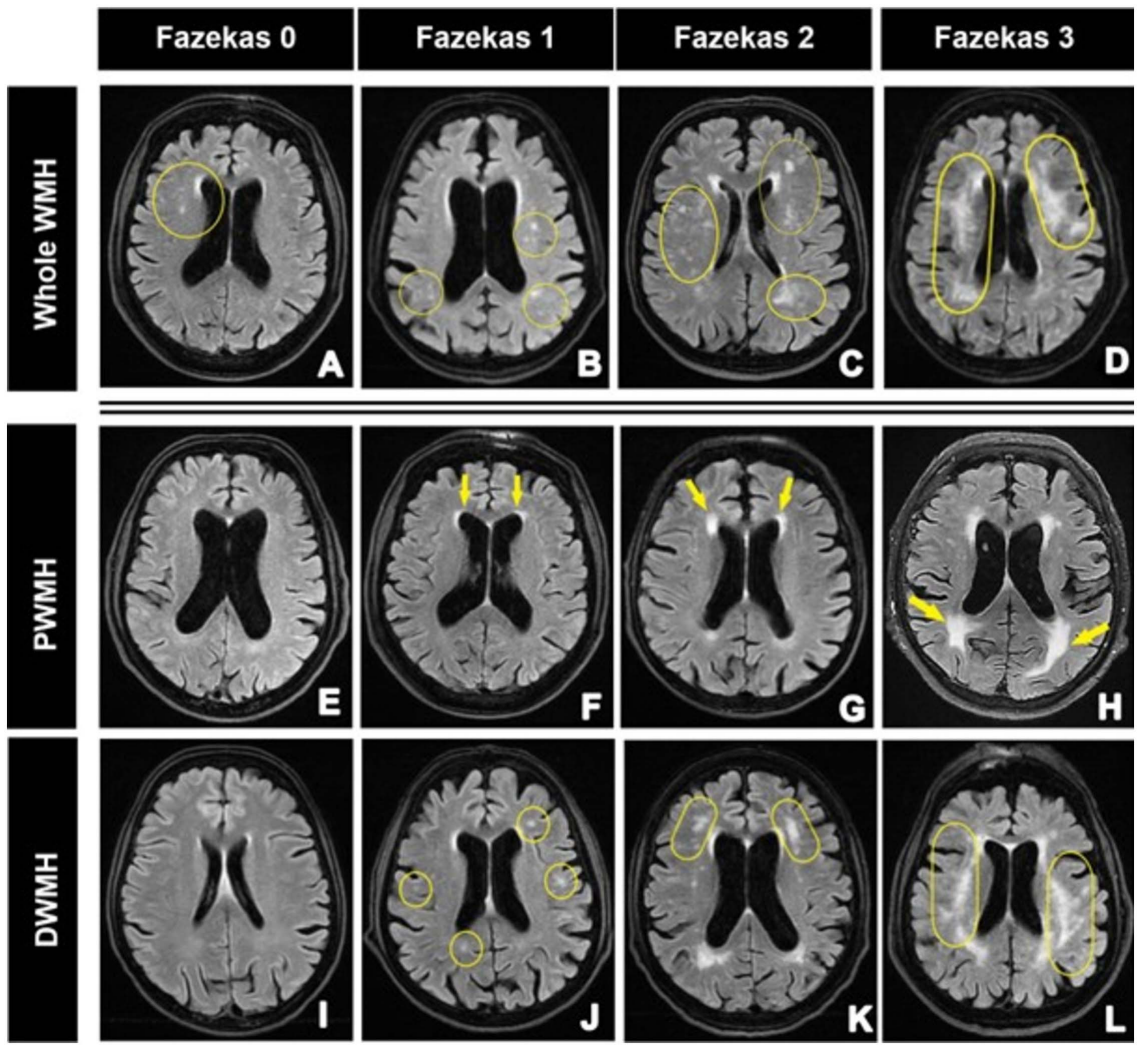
Axial FLAIR images of different patients, mostly with Alzheimer’s disease and vascular dementia clinical presentations (aged between 55 and 87 years) show Fazekas scales. The first row: whole WMHs: (A) single WMH (circled yellow); (B) multiple WMHs (circled yellow); (C) WMHs that start to bridge (circled yellow); (D) large confluent WMHs (circled yellow). The second row: PWMH: (E) absent WMH; (F) “cap and bands” (yellow arrows); (G) smooth periventricular hallo (yellow arrows); (H) PWM extends into DWM (yellow arrows). The third row: DWMH: (I) absent WMHS; (J) punctate foci (circled yellow); (K) WMHs that start to confluent (circled yellow); (L) large confluent WMHs (circled yellow). FLAIR: Three-dimensional T2-weighted fluid-attenuated inversion-recovery imaging; WMH: White matter hyperintensities; DWMH: Deep white matter hyperintensities; PWMH: Periventricular white matter hyperintensities.
